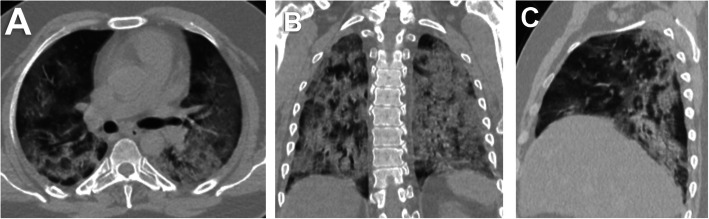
A 44-year-old male patient. A axial, B coronal, and C sagittal chest CT images show bilateral diffuse mainly peripheral ground-glass opacities and consolidation with severe pulmonary involvement (50-75%) corresponds to current chest CT score 19 and simple chest CT score 4 (severe affection) and. The patient survived
